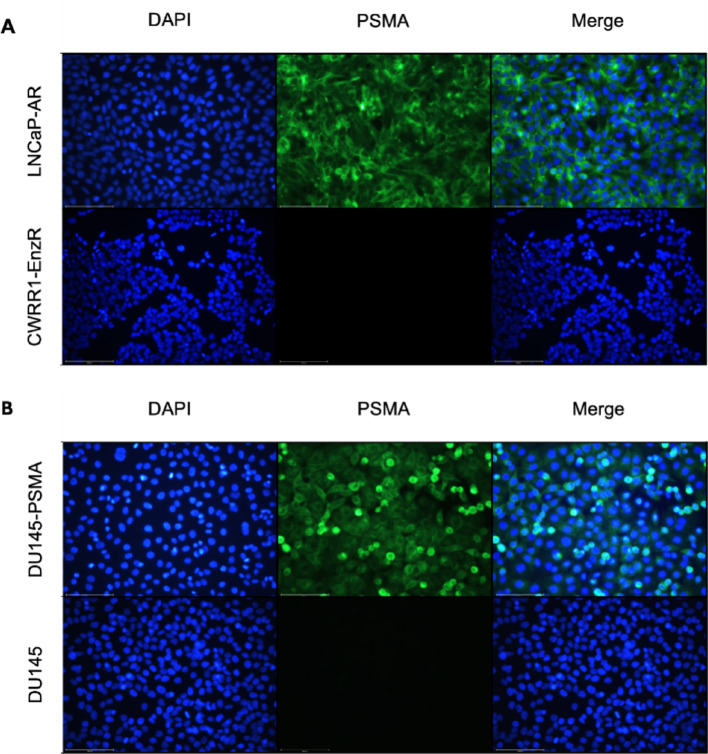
Immunocytochemical imaging of PSMA expression in cell line pairs. Images are paired as (A) the LNCaP-AR (PSMA-positive) and CWRR1-EnzR (PSMA-negative) pair, and (B) the isogenic DU145-PSMA (PSMA-positive) and DU145 (PSMA-negative) pair
